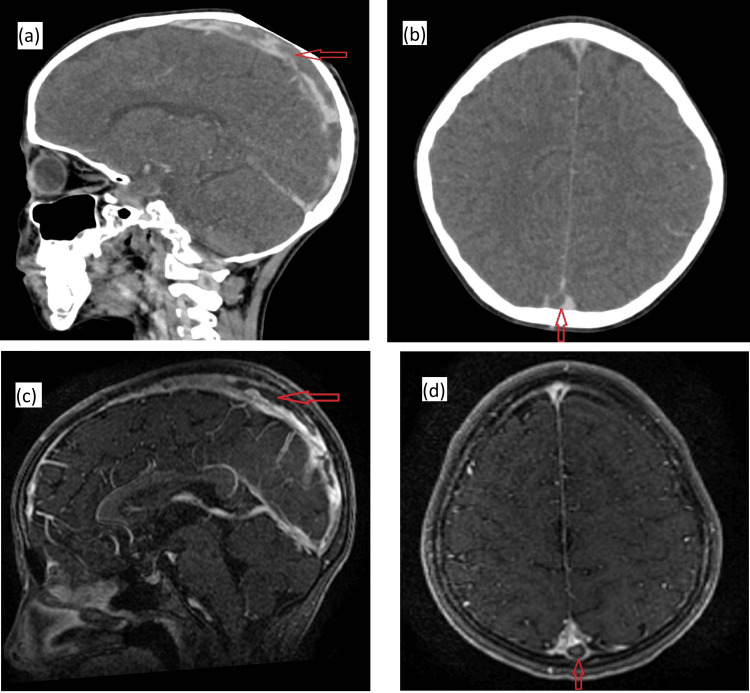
(a) Sagittal CT image with contrast injection showing a hypodense area within the superior sagittal sinus (red arrow), consistent with cerebral thrombophlebitis. (b) Axial CT image with contrast injection showing a filling defect within the superior sagittal sinus corresponding to the empty delta sign (red arrow). (c) Sagittal MRI (Fast Spoiled Gradient Echo (FSPGR) sequence) demonstrating a hypointense signal within the superior sagittal sinus, compatible with thrombosis. (d) Axial MRI (FSPGR sequence) highlighting the empty delta sign (red arrow).
